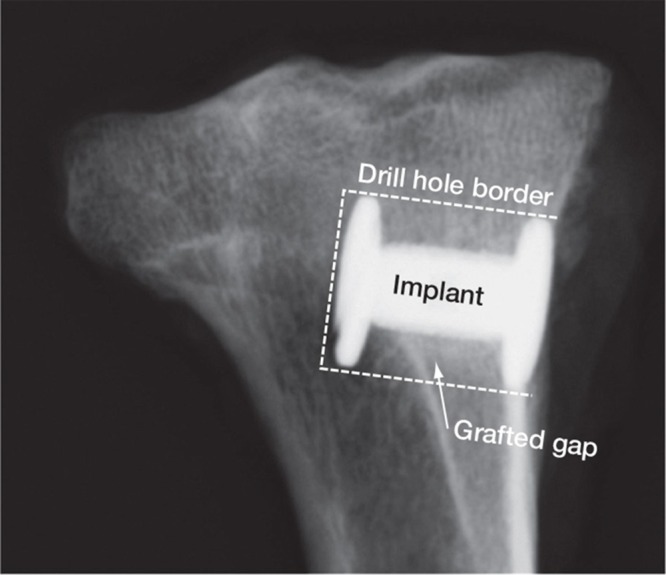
Gap-implant in the proximal tibia. Radiograph taken post mortem.
